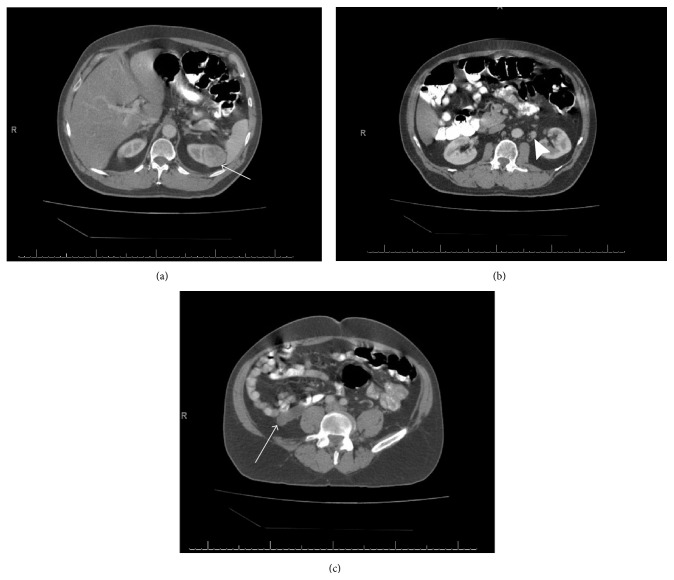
CT scans reveal a partially enhancing mass ((a) arrow) located on the upper pole of the left kidney. The presence of para-aortic lymphadenopathy ((b) arrowhead) as well as a prominent appendiceal mass ((c) arrow) was also noted.
